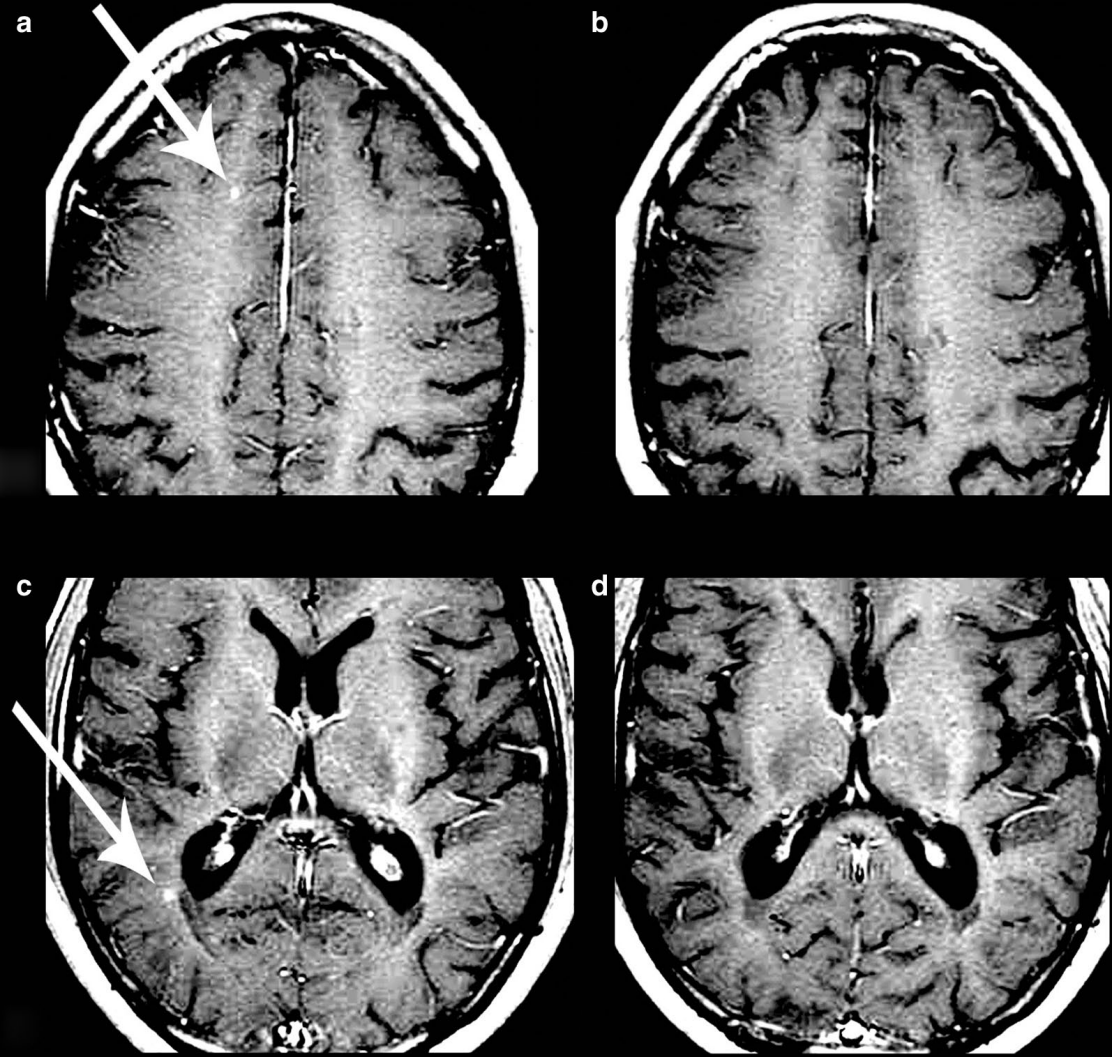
Changes in magnetic resonance imaging (MRI) before and after treatment. Gadolinium-enhanced MRI scans of the brain for one subject before (a and c) and after (b and d) treatment. Lesions of interest are indicated by a white arrow. b Interval resolution of a lesion in the right frontal juxtacortical white matter (a). d Interval resolution of a lesion in the right periatrial white matter (c). Names and other personal information have been edited out of the images
